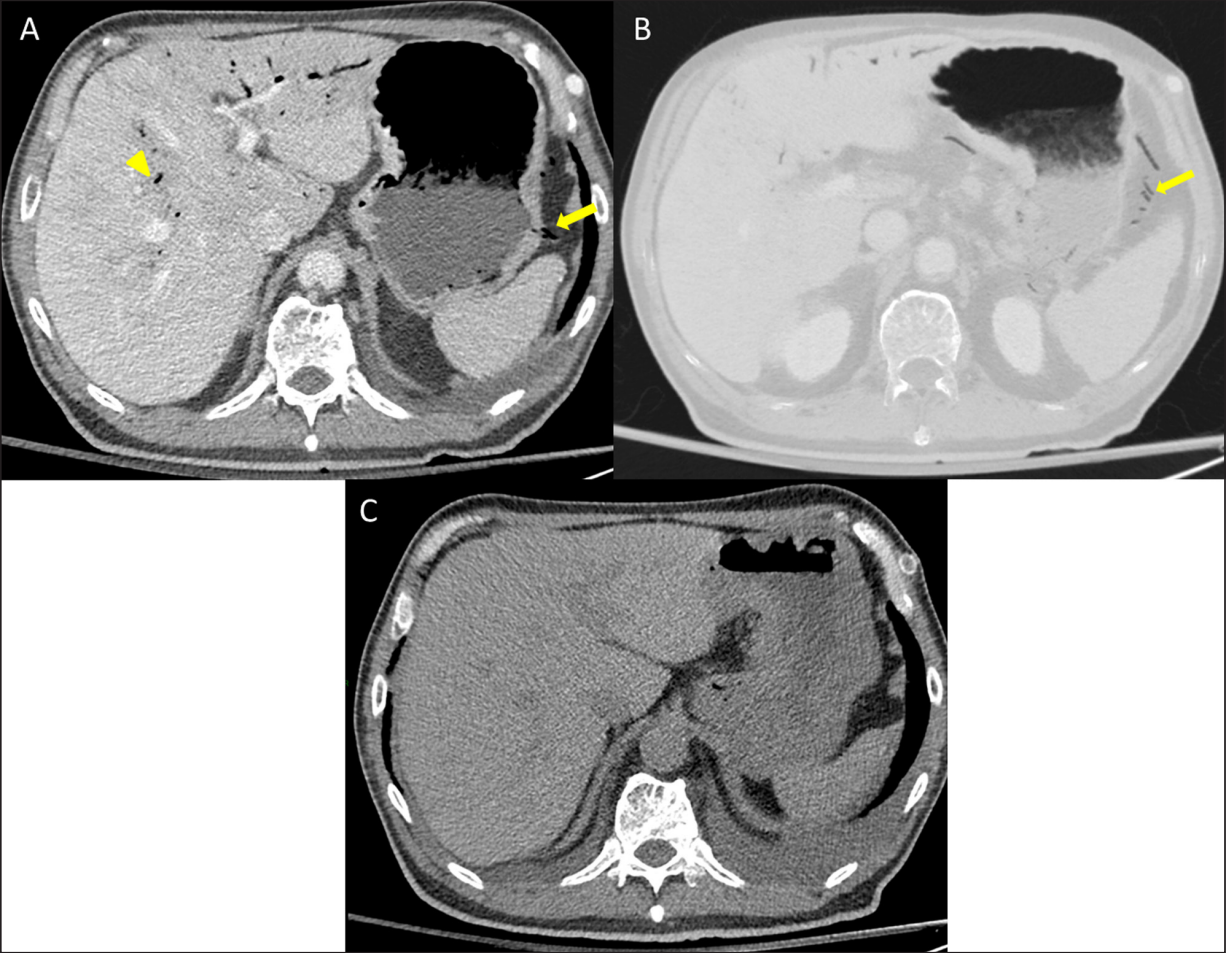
A) Axial CT image in portal phase showing portal venous gas (arrowhead) and gas in perigastric veins (arrow). Note the liver parenchyma hypoperfusion around the aeroportia and normal enhancement of gastric wall. B) Minip reconstruction showing gas in perigastric veins. C) Unenhanced axial CT image performed 12 hours after the vomiting episode showing complete resolutions of venous gas (portal and perigastric).
